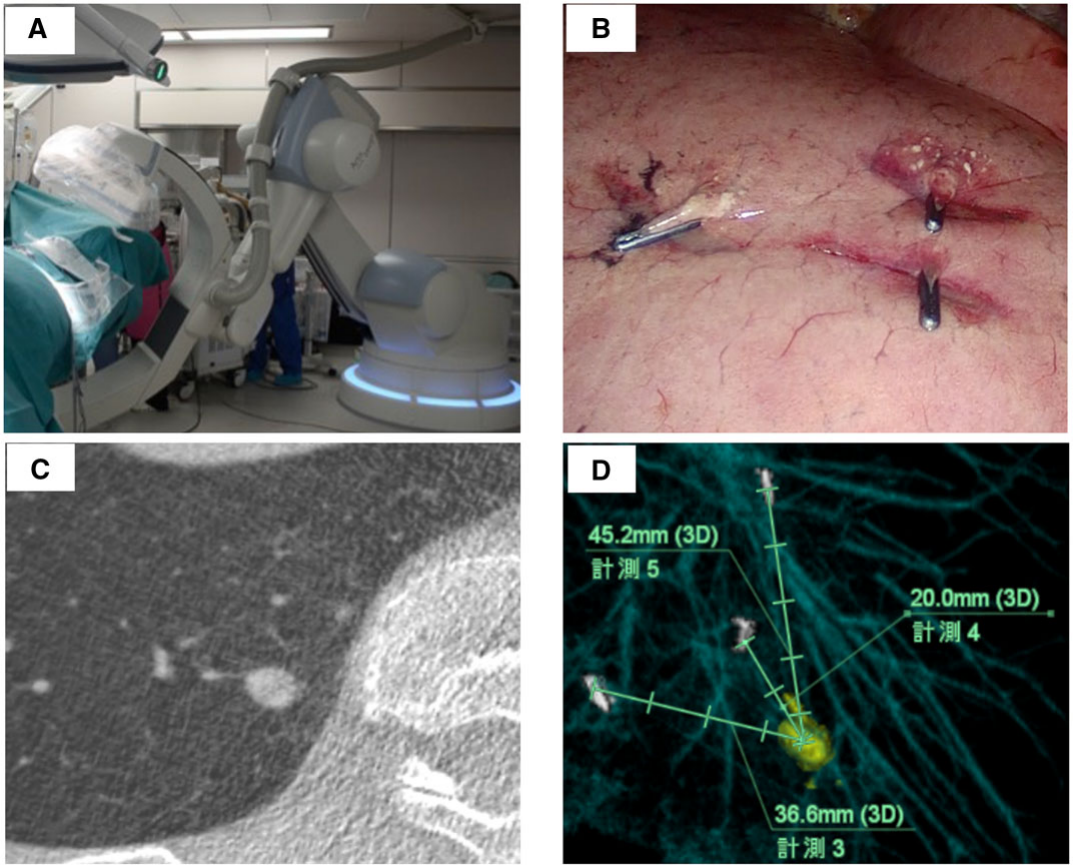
Procedure for localization of pulmonary tumor by cone-beam CT. (A) In a hybrid operating room, we used the cone-beam CT system Artis zeego® (Siemens, Bayern, Germany) and analyzed tumor location using a Ziostation 2® (Ziosoft, Tokyo, Japan). (B) Four surgical clips are attached to the visceral pleura around the suspected location of the referred tumor. (C) CBCT CT image of a deep solid-type tumor. (D) Analysis of the location of the referred tumor. CBCT: cone-beam computed tomography; CT: computed tomography.
